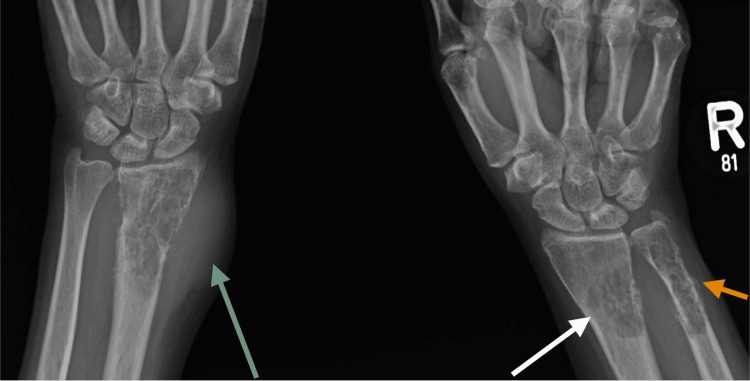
Bilateral wrist radiography in anteroposterior view Seen are lytic bone lesions with internal tribulation (white arrow) with moth-eaten appearance, involving the distal radius bilaterally and right ulna with cortical bone erosion and early destruction associated with periosteal reaction (orange arrow) and soft tissue component on the left wrist (green arrow). No fracture line or other similar lesions were observed.
